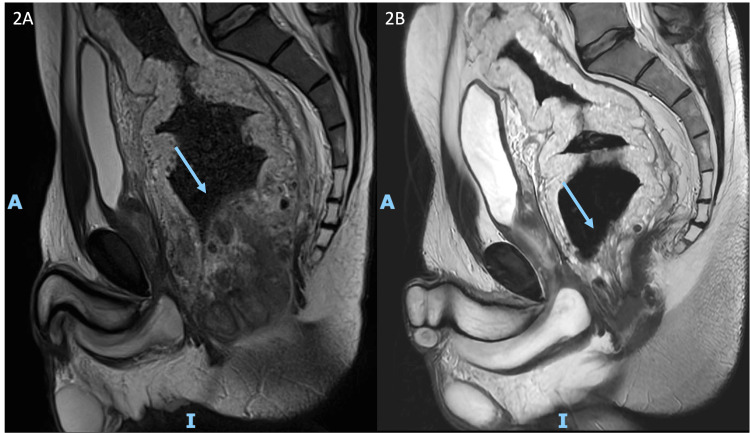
Contrast-enhanced MRI of the pelvis (a) MRI showing venous lakes in the rectum and anus (arrow). (b) MRI after six months with significant reduction of venous lakes
